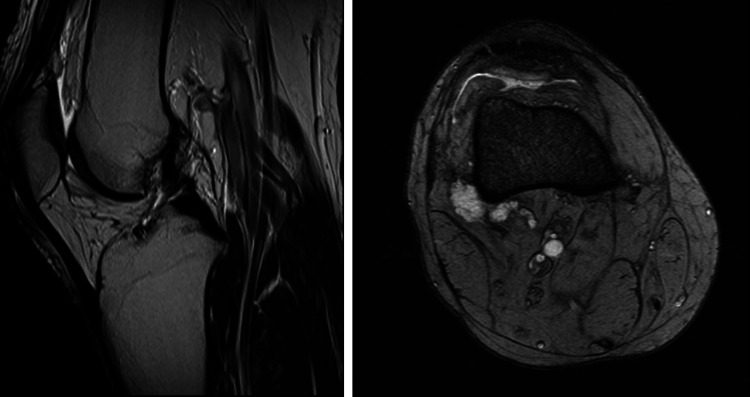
T2-weighted sagittal MRI of the right knee demonstrating the absence of a characteristic hyperintense necrotic center or surrounding inflammatory edema within the superolateral suprapatellar pouch corresponding to the necrotizing granuloma identified intraoperatively.
